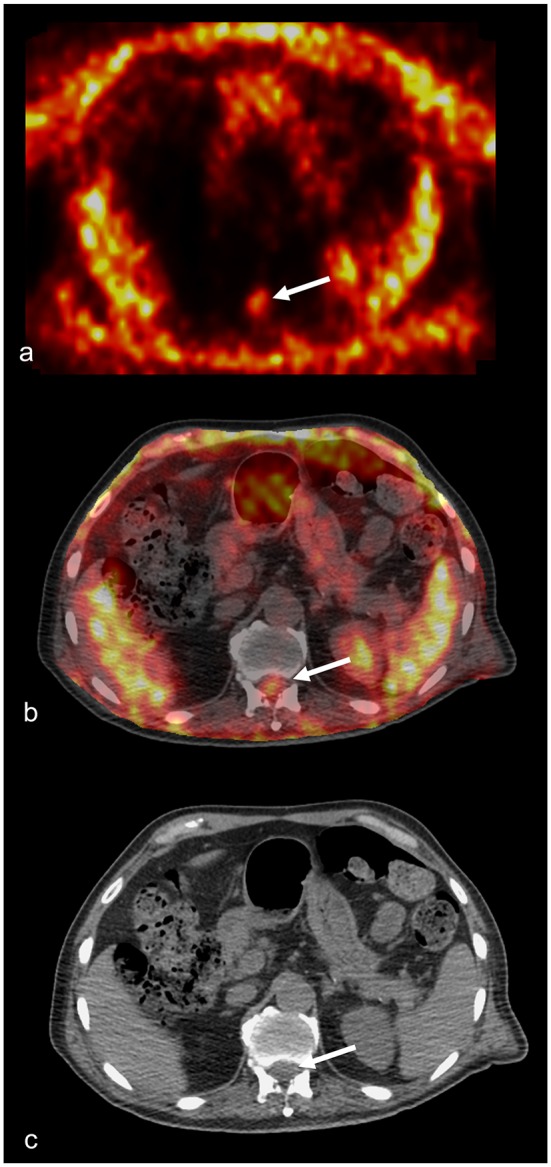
^18^FDG-PET and CT scan of patient no. 3. (a)
